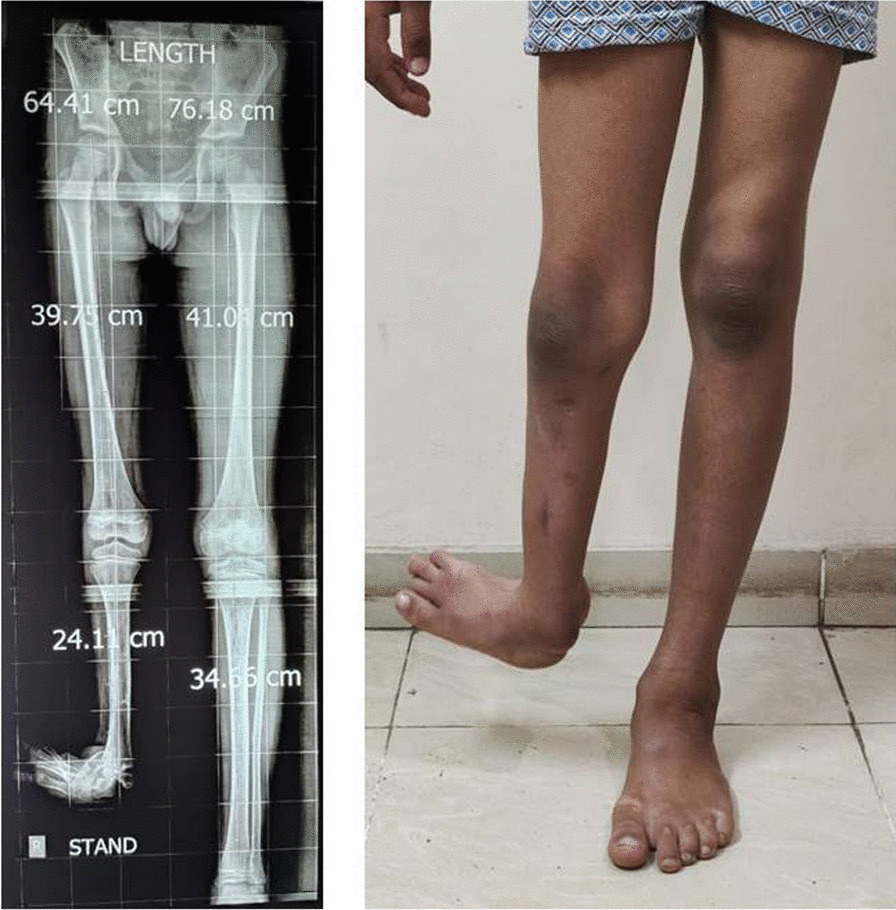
A full-length standing X-ray scanogram was obtained in all cases, with blocks under the short limb to equalize the length
